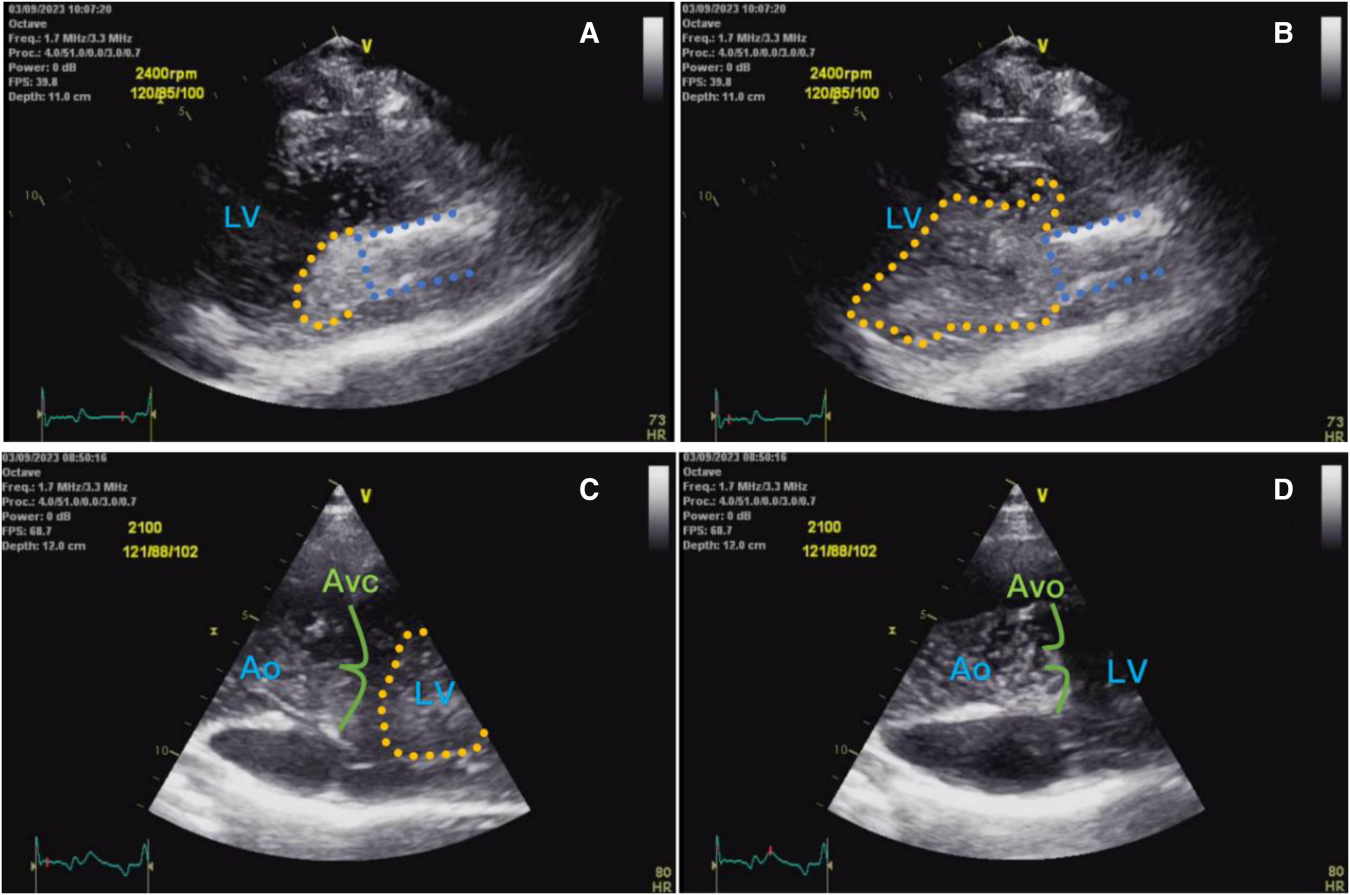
(A) Shows ultrasound left ventricular long-axis view of a small number of bubbles (yellow dashed area) entering the left ventricle from the pump inflow tube (blue dashed area) during early diastole; (B) shows a large number of bubbles entering the left ventricle from the inflow tube at end-diastole; (C) shows a large number of bubble clusters in the left ventricle during diastole, with a moderate number of bubbles in the ascending aorta; and (D) shows a large number of bubbles in the left ventricle during systole entering the ascending aorta via the aortic valve.
